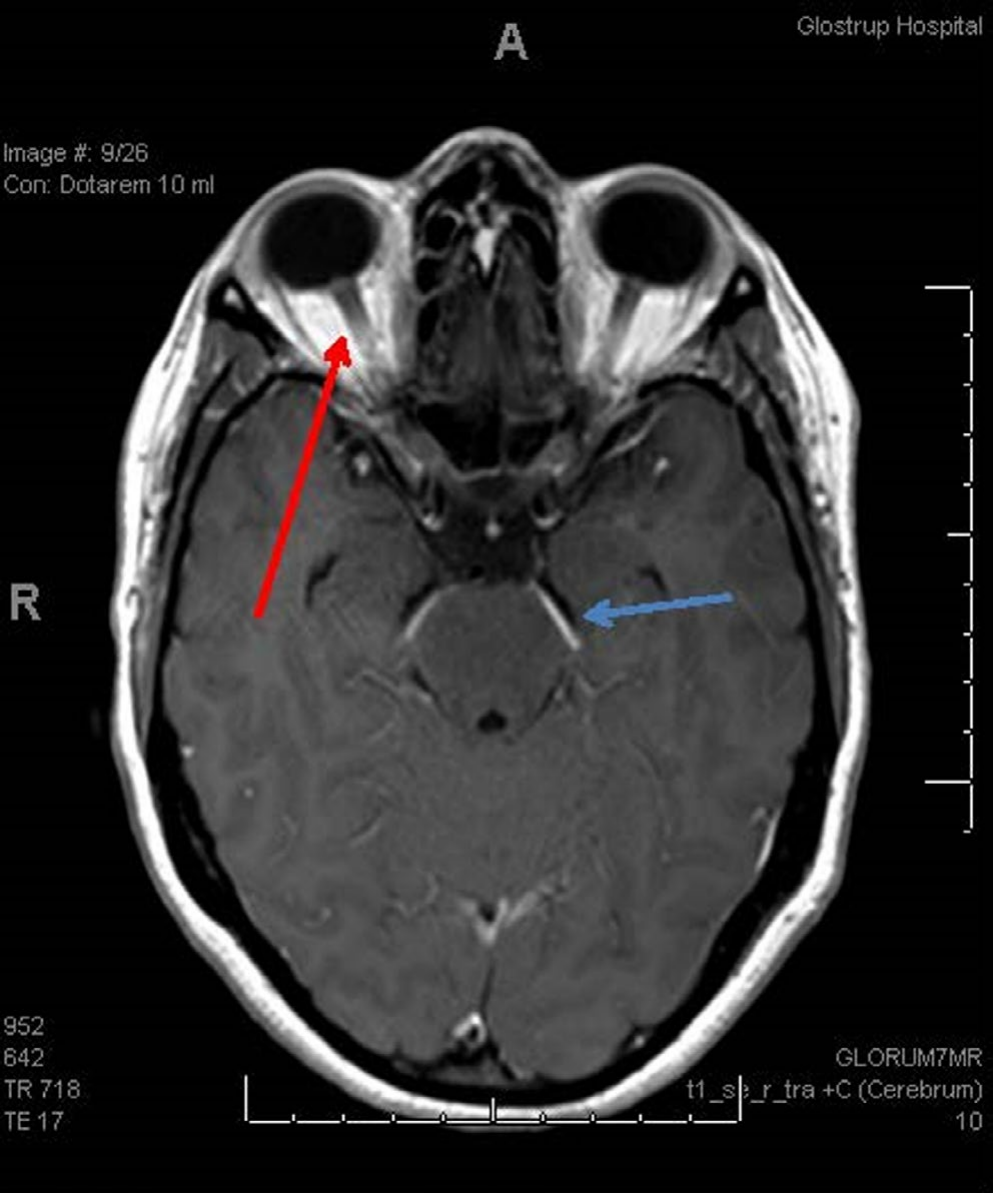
MRI of cerebrum with gadolinium contrast, axial picture. Red arrow showing papilledema and blue arrow showing meningeal enhancement
